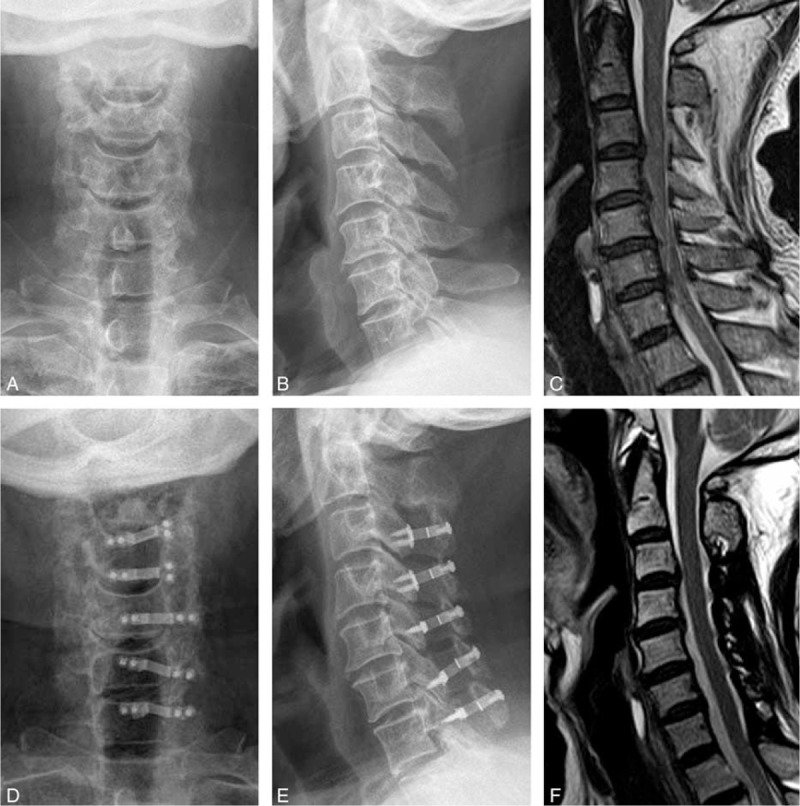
A 65 years old man patients. Preoperative X-ray plain film and middle sagittal film of magnetic resonance showed the cervical spine canal stenosis and the spinal cord compression (A–C). Preoperative X-ray plain film and middle sagittal film of magnetic resonance showed cervical canal expansive, spinal cord decompression and good miniplate fixation (D–F).
